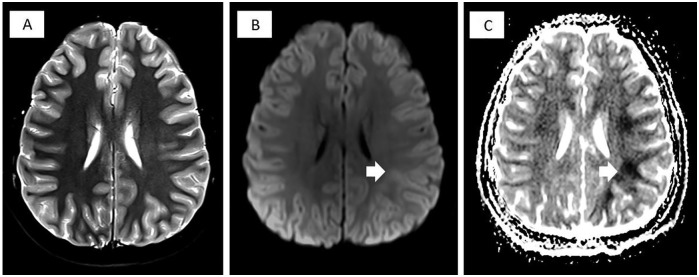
Brain magnetic resonance imaging of a patient with methotrexate neurotoxicity
